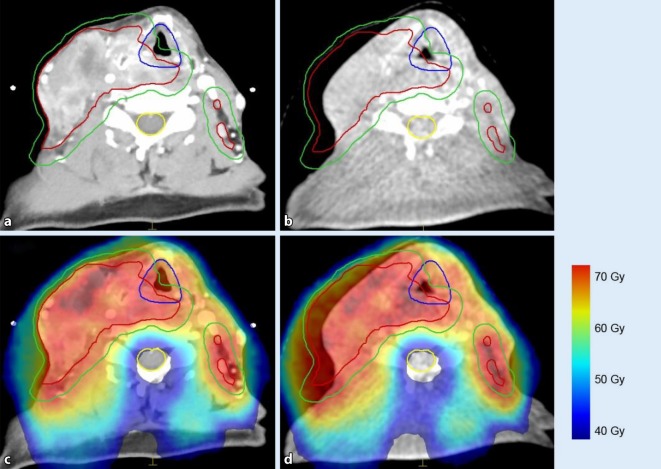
Image-guided radiotherapy (RT) for head and neck cancer. Planning computed tomography (a) overlaid with dose (c); same patient in week 5 of treatment (b, d). Although the treated lymph nodes evidently regressed (b), dose coverage is maintained even without adaptation of the treatment fields in this photon-based rotational arc RT (d). Gross tumor volume: *red contour*; planning target volume: *green contour*; larynx: *blue contour*; spinal canal: *yellow contour*
